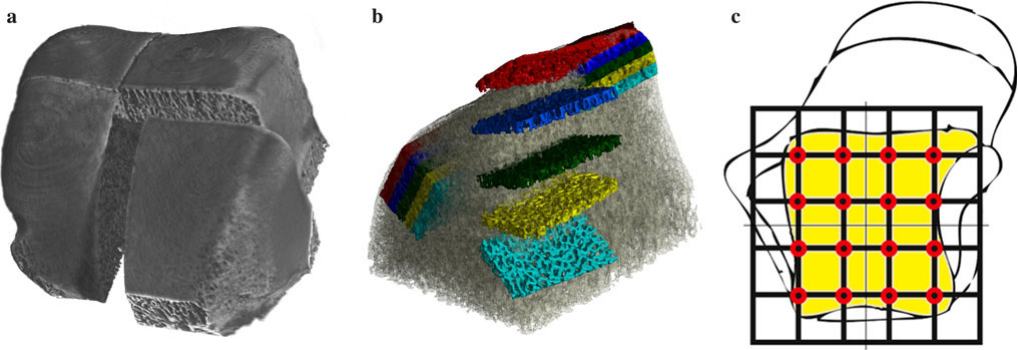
Definition of measured zones: a 3D reconstruction of the Micro CT data of an initially quartered talar trochlea. b Representation of the 5 × 1 mm thick layers as five separate volume elements. Each talar quarter from Fig. 1a was divided into four areas, one of them shown here as an expanded view for better visualization. c The 4 zones from b on the 4 talar quarters from a yields 16 zones in 5 layers, thus a total of 80 separate volume elements
